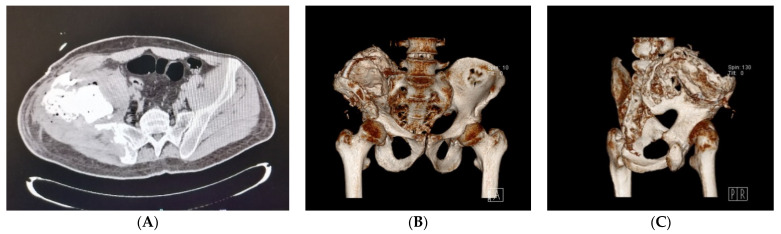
Postoperative CT scan (A) and 3D reconstruction (B,C) of the pelvis.
